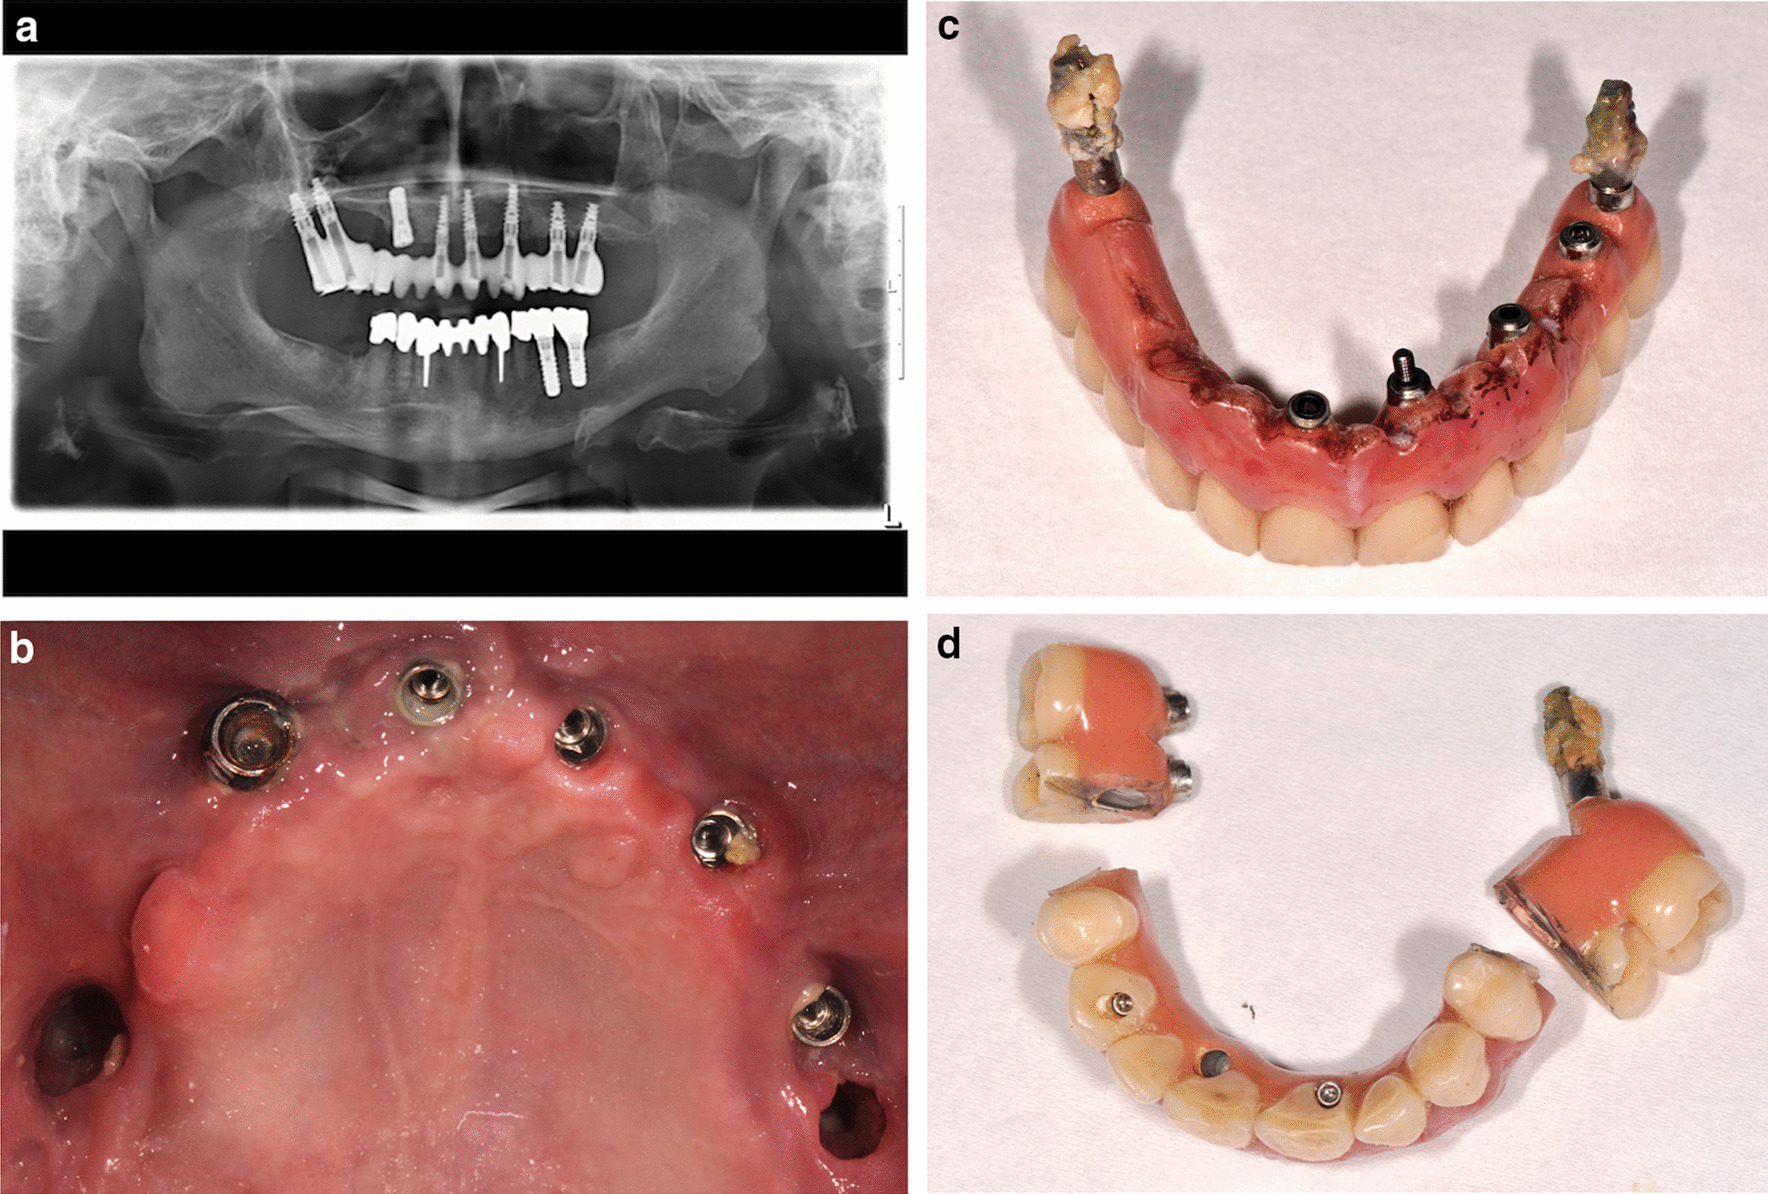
a Panoramic radiograph. b Initial intraoral situation. c Removed prosthesis. d New adjustment configuration of the prosthesis
